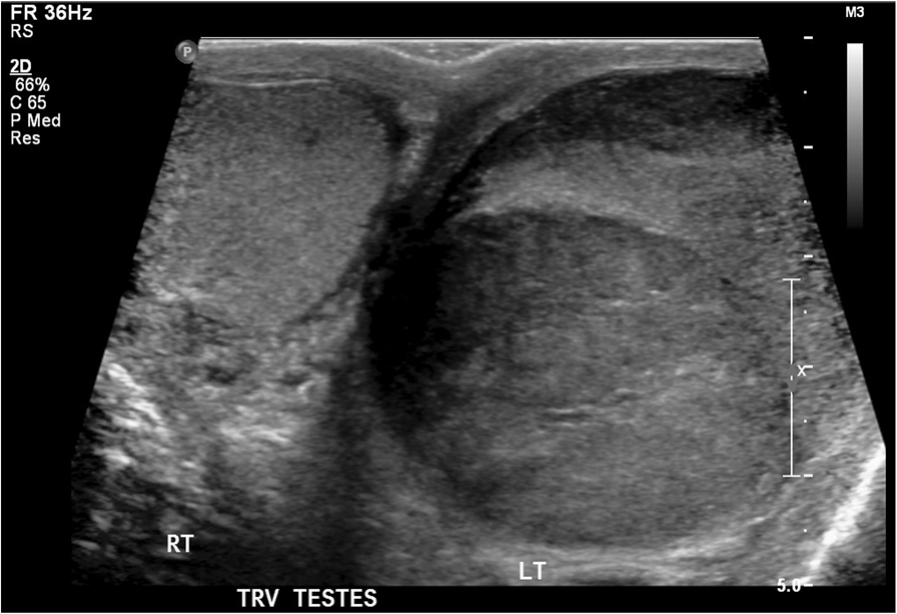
Ultrasound image of the patient’s left testis. The left testis is replaced by a large heterogenous mass
